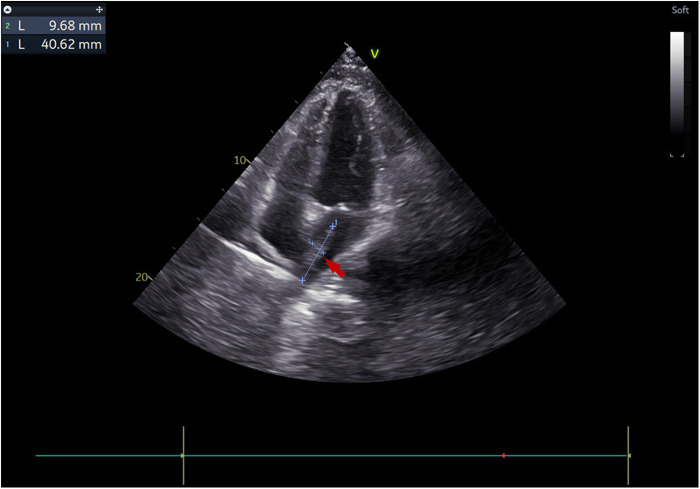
Transthoracic echocardiography visualized a 4 × 1 cm pedunculated vegetation (arrow) in the left atrium in the apical four-chamber view.
